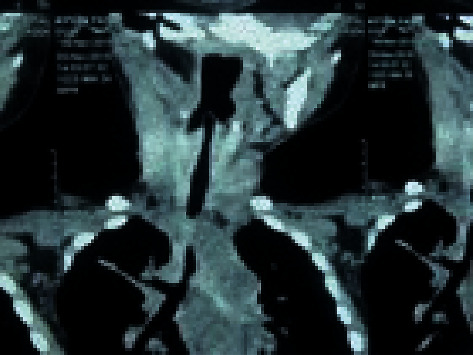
Preop coronal CT appearance in a child with acquired subglottic stenosis.
